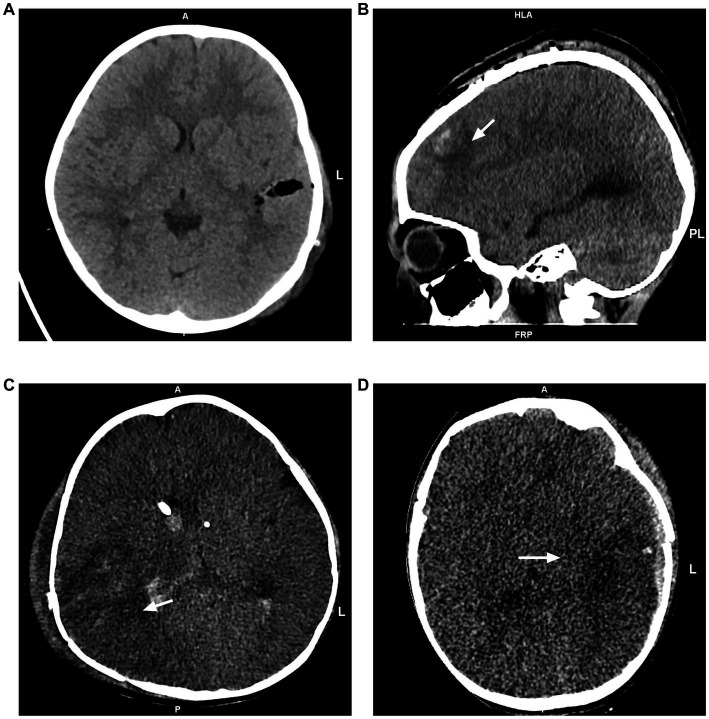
Postoperative head CT images. (A) No edema was observed. (B) Focal edema limited to the left frontal lobe. (C) Unilateral edema in the right temporal, occipital, and parietal lobes. (D) Global edema in the left cerebral hemisphere.
